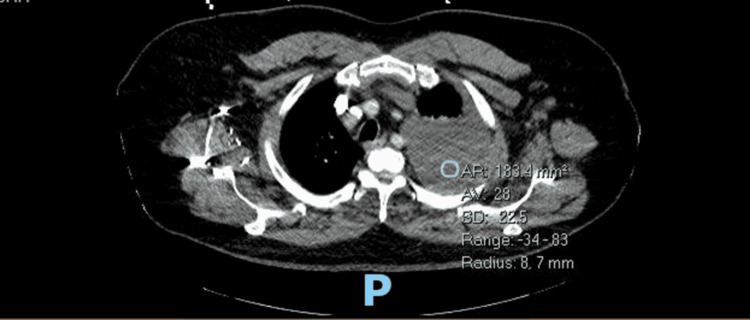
A large, left-sided pleural effusion and a small pericardial effusion seen on CT chest
